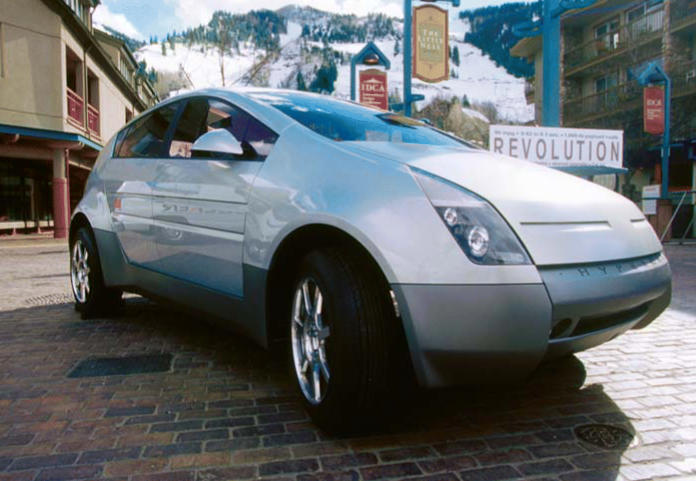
Stopping traffic The 2000 Hypercar Revolution models the virtual design of a midsize SUV. The carbon fiber body makes it lighter, safer, and—at 114 mpg with a fuel cell—far more fuel-efficient than traditional cars.
